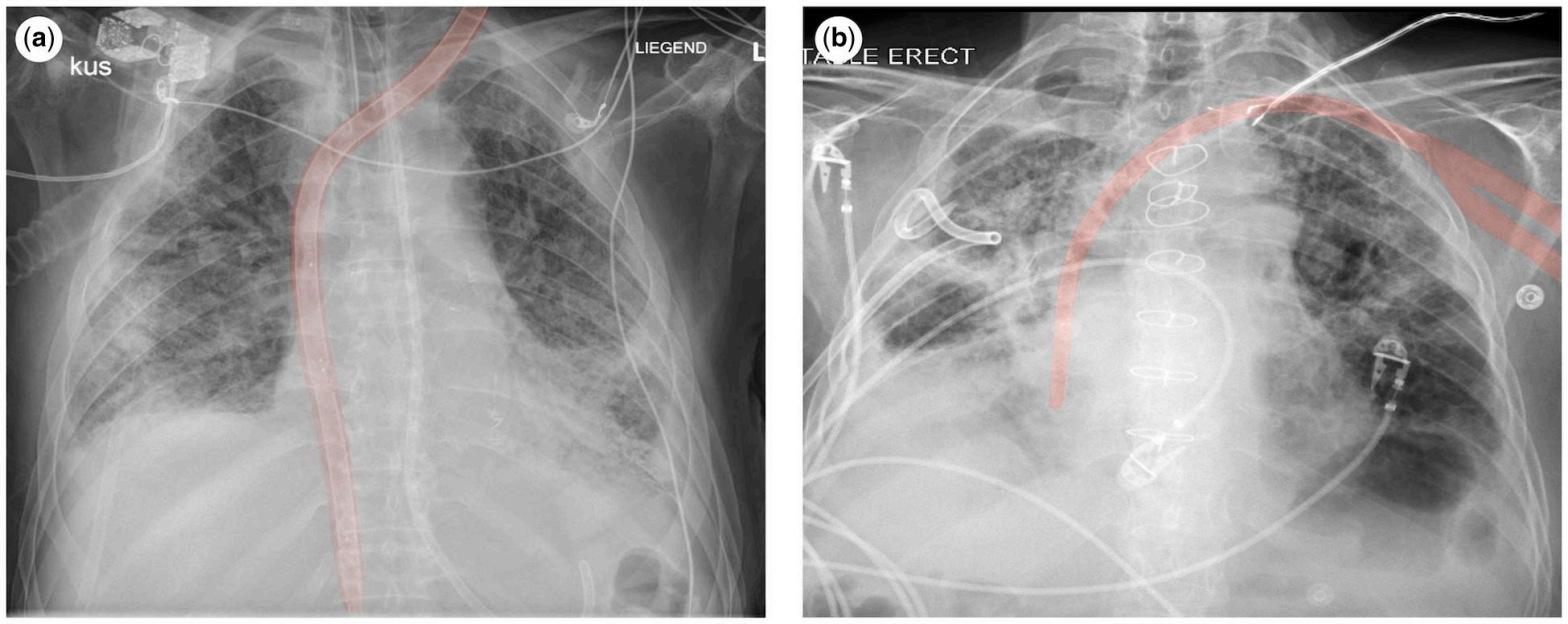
Chest X-ray of a 28 FR Crescent cannula inserted via the LIJV in an 58-year-old patient with hypercapnic and hypoxemic respiratory failure and thrombosis of the RIJV (a) and chest X-ray of an 19 FR Avalon cannula inserted via the left subclavian vein in an 61-year-old patient with hypercapnic respiratory failure (b). FR: French; LIJV: left internal jugular vein; RIJV: right internal jugular vein.
